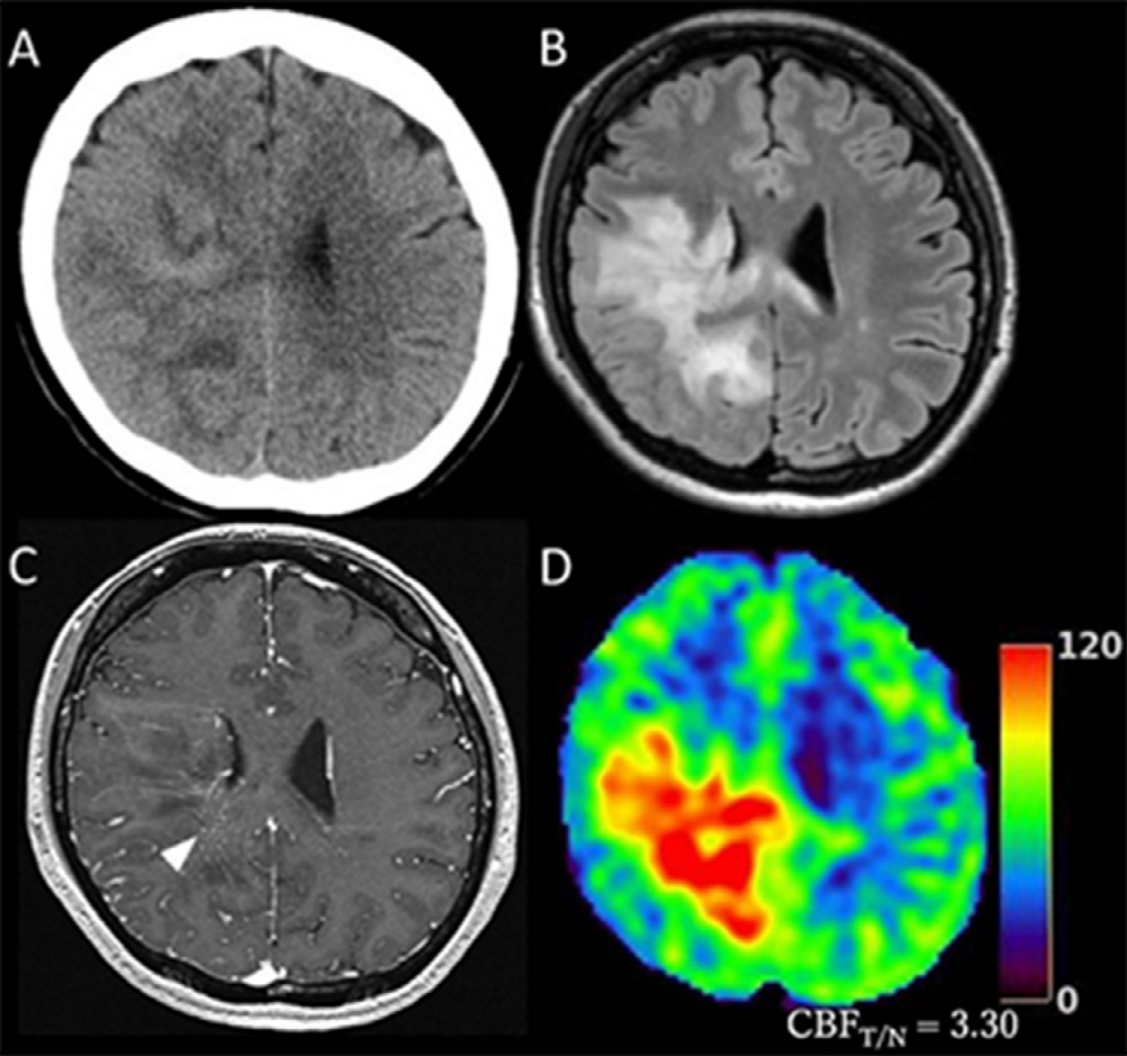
Neuroimaging on admission to our hospital. (A) Non-contrast CT. (B) FLAIR. (C) Gd-T1WI. (D) ASL. Arrowhead represents slight enhancement.
